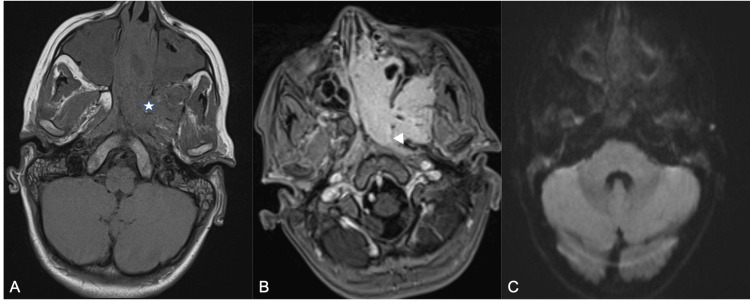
Contrast-enhanced MRI highlighting local invasion of adjacent structures with no restriction on DWI imaging. At axial non-contrast T1-weighted images (A). JNA is predominantly isointense arrising from the spheno-palatine foramen (asterisk), with intense and heterogeneous enhancement after contrast injection (B) and in this case invasion of the adjacent pterygoid muscle (arrow head). At axial DWI (C), JNA does not present a high signal.
